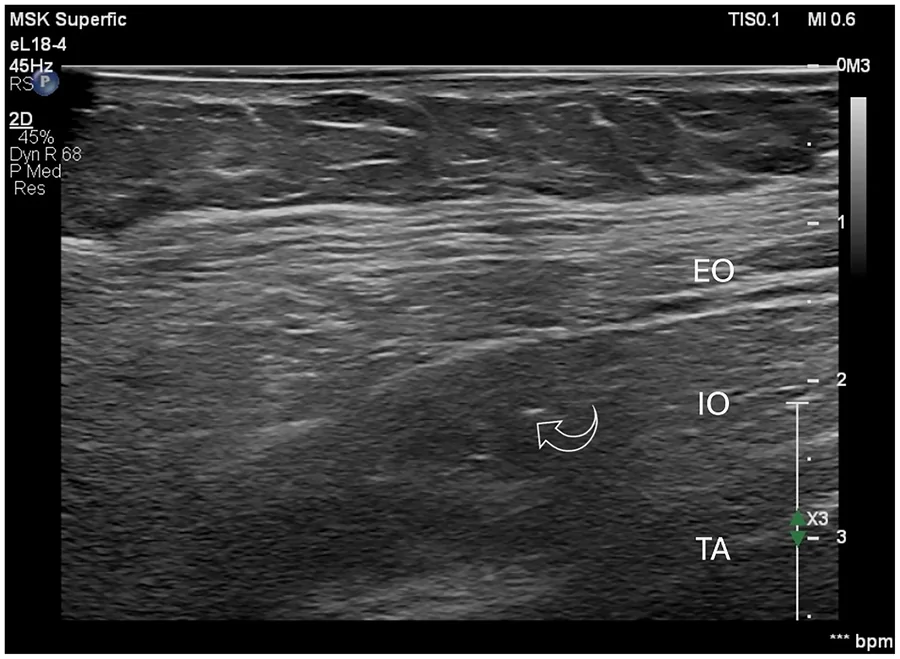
Ultrasound-guided botulinum toxin A injection. Ultrasound image demonstrating the injection needle (arrow) positioned within the transversus abdominis (TA) muscle, indicates the needle tip, and the solution is faintly visible at the tip. The three lateral abdominal wall muscle layers are identified: external oblique (EO), internal oblique (IO), and transversus abdominis (TA).
